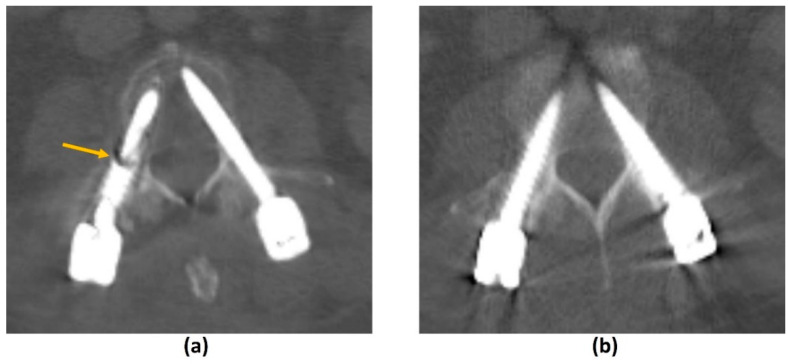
Axial CT-images in a 53-year-old patient with spinal implants with (a) and without (b) a post-processing metal artifact reduction (MAR) algorithm. While there is a noticeable reduction in metal-related artifacts with the MAR algorithm, there is an apparent linear lucency (solid arrow) across the right screw at the pedicle region. This was a new streak artifact inadvertently produced by the MAR algorithm and comparison with the non-MAR images is important to avoid mistaking these new artifacts as implant fractures.
